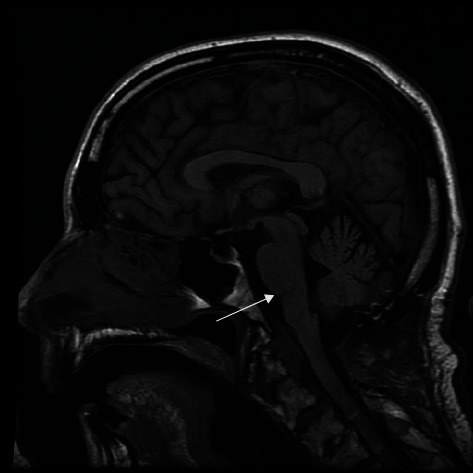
Postoperative MR brain T1 FLAIR sagittal image demonstrates resolution of the cervicomedullary compression and caudal medullary cyst.
